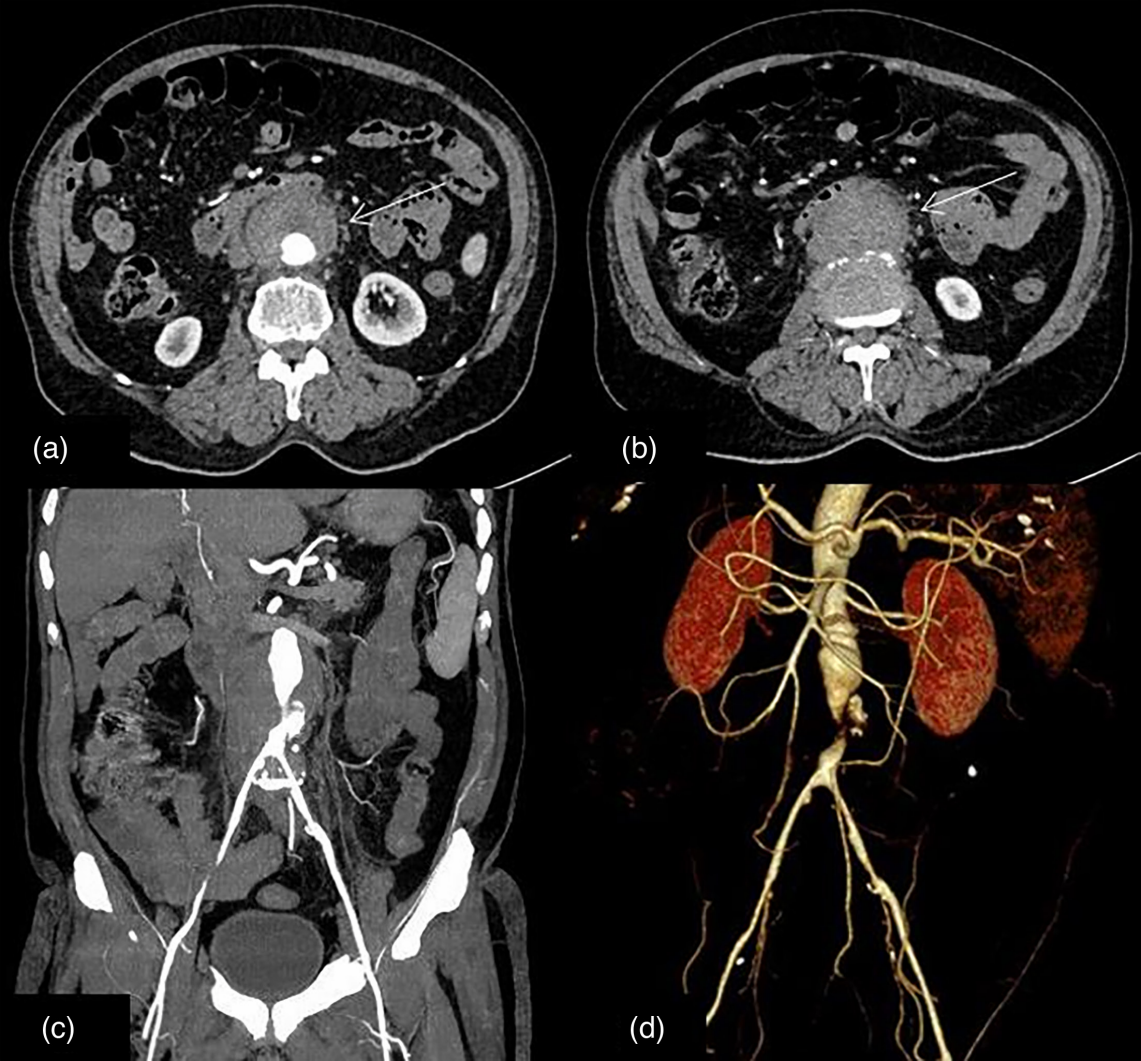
CT abdominal angiograms in a 70-year-old gentleman showing a ruptured infra-renal abdominal aortic pseudoaneurysm (white arrow in a, and c). His blood cultures were positive for *Salmonella paratyphi A*. Repeat CT angiogram after antibiotic therapy showed thrombosis of the pseudoaneurysm (white arrows in b, and d).
